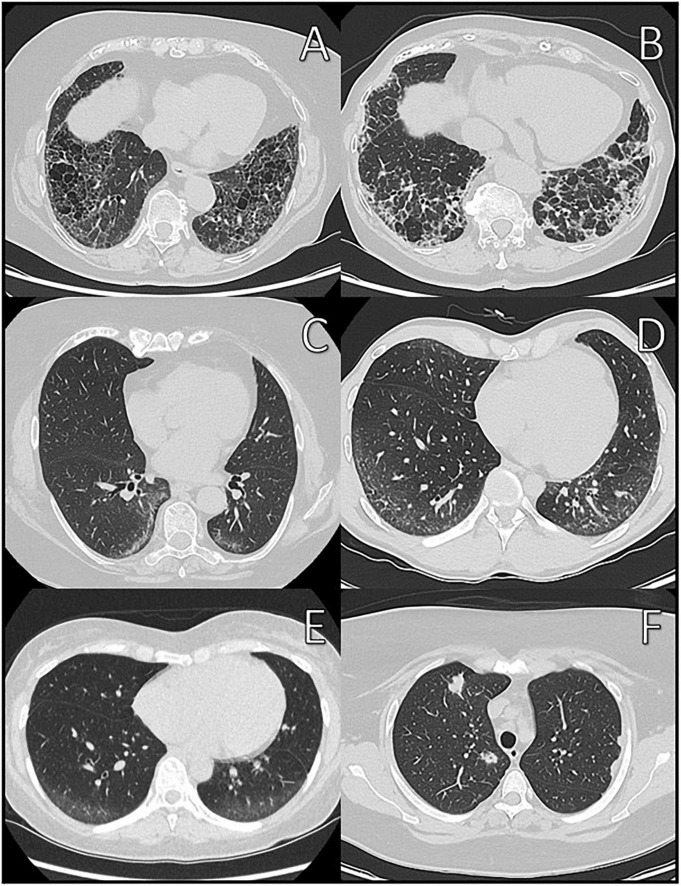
Different CT-patterns in patient with IRD at initial diagnosis. (A) Usual interstitial pneumonia (UIP) in polymyositis; (B) probable UIP in systemic sclerosis; (C,D) non-specific interstitial pneumonia (NSIP) in Sharp syndrome; (E) pure ground-glass opacities in Sharp syndrome; and (F) granuloma/proliferations in granulomatosis with polyangiitis.
